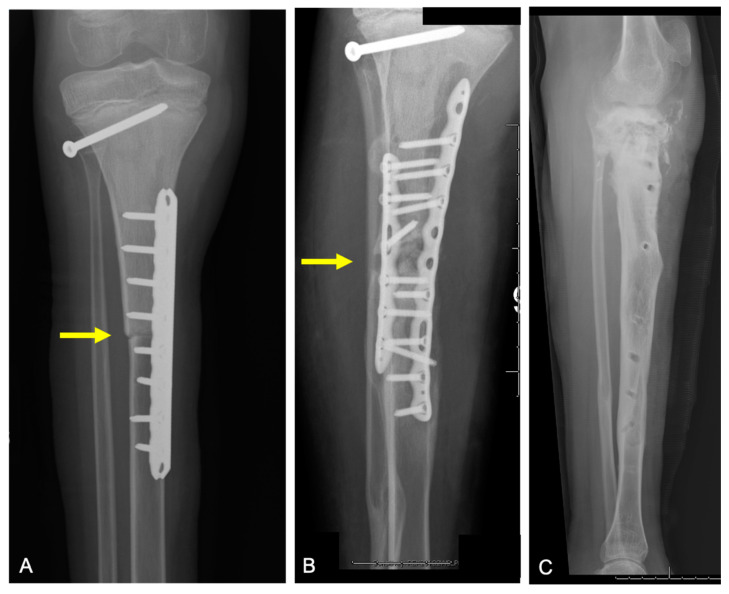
Plain radiographs in a patient with proximal tibial osteoarticular allograft placed after osteosarcoma resection, experiencing atrophic allograft nonunion (type 2B failure) and subsequent hardware loosening. (A) Initial radiograph 1.5 years after allograft placement demonstrates concern for nonunion with formation of fibrous tissue-filled gap between allograft and native bone (yellow arrow), and additional clinical concern for chronic infection. Allograft revision was performed, and (B) two-year follow-up images demonstrate persistent nonunion (yellow arrow). Subsequently, cable bone transport techniques were utilized to bridge the bone defect and achieve adequate limb length. (C) Radiograph after removal of all hardware (6 years after initial tumor resection) demonstrates solid regenerated bone and remodeling of the tibial shaft but a collapse of the graft articular surface.
